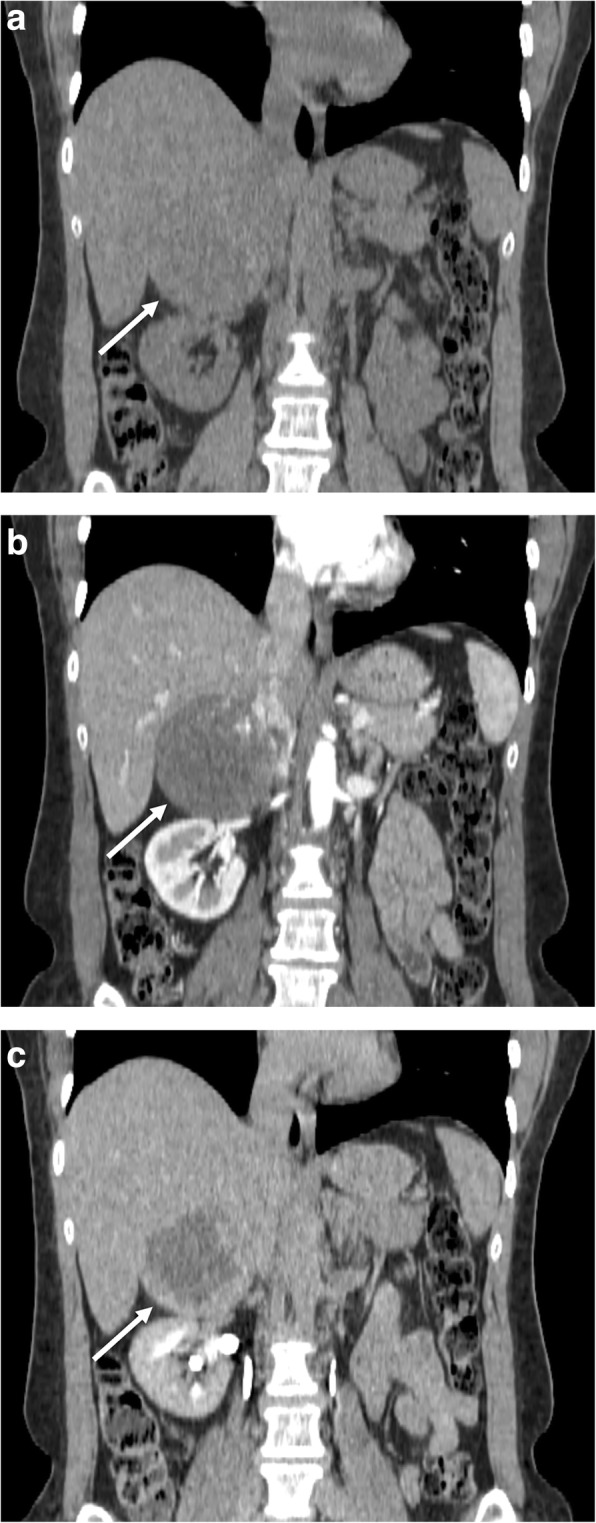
Right adrenal hemangioma in a 49-year-old woman. Coronal reformatted CT images before (a) and after contrast injection in arterial (b) and late phase (c) show a well-encapsulated large right adrenal lesion (arrow) showing peripheral contrast enhancement with progressive centripetal filling and a hypodense center
